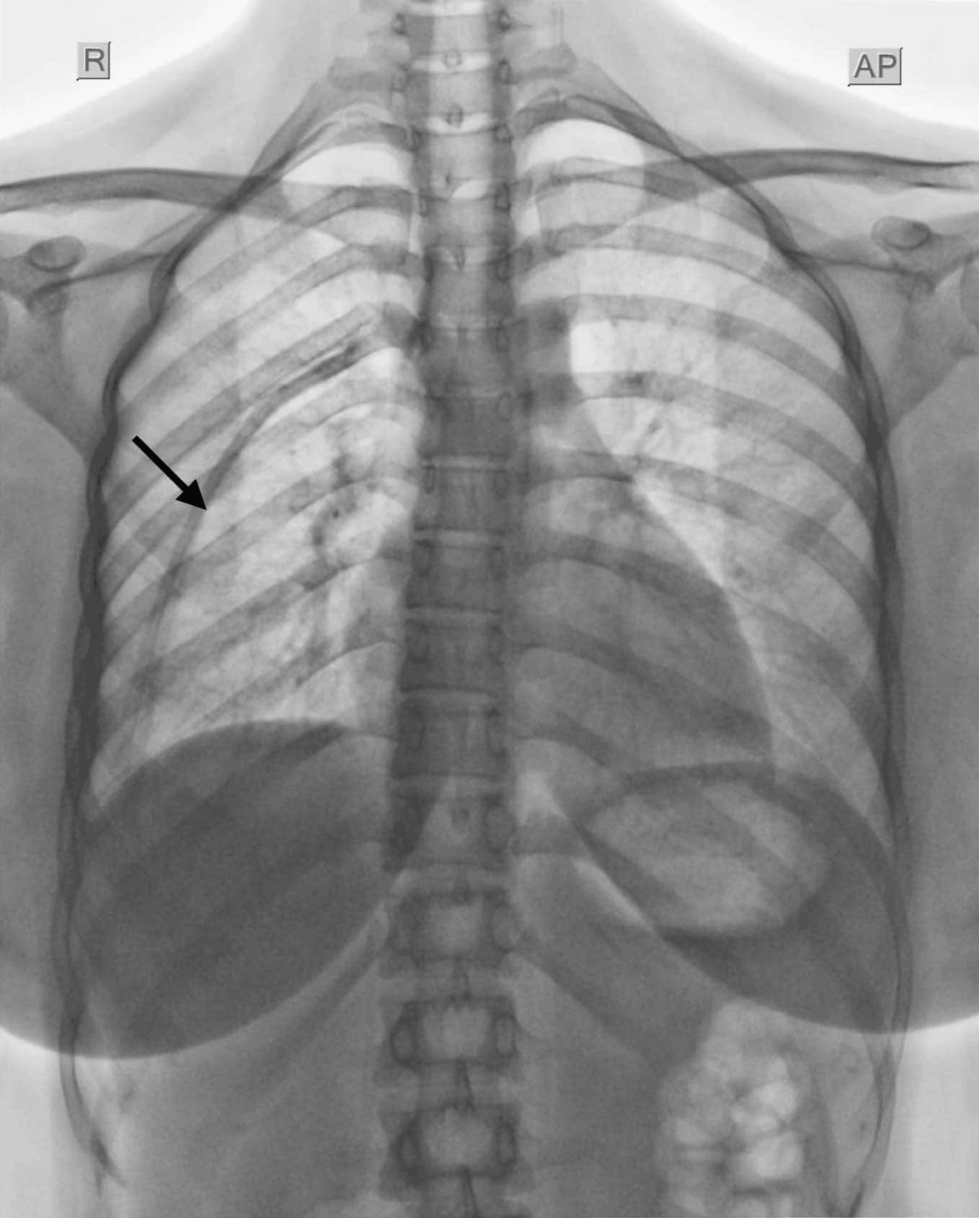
An enhanced chest radiograph showing evidence of a bifid intrathoracic fifth rib in the right hemithorax.
